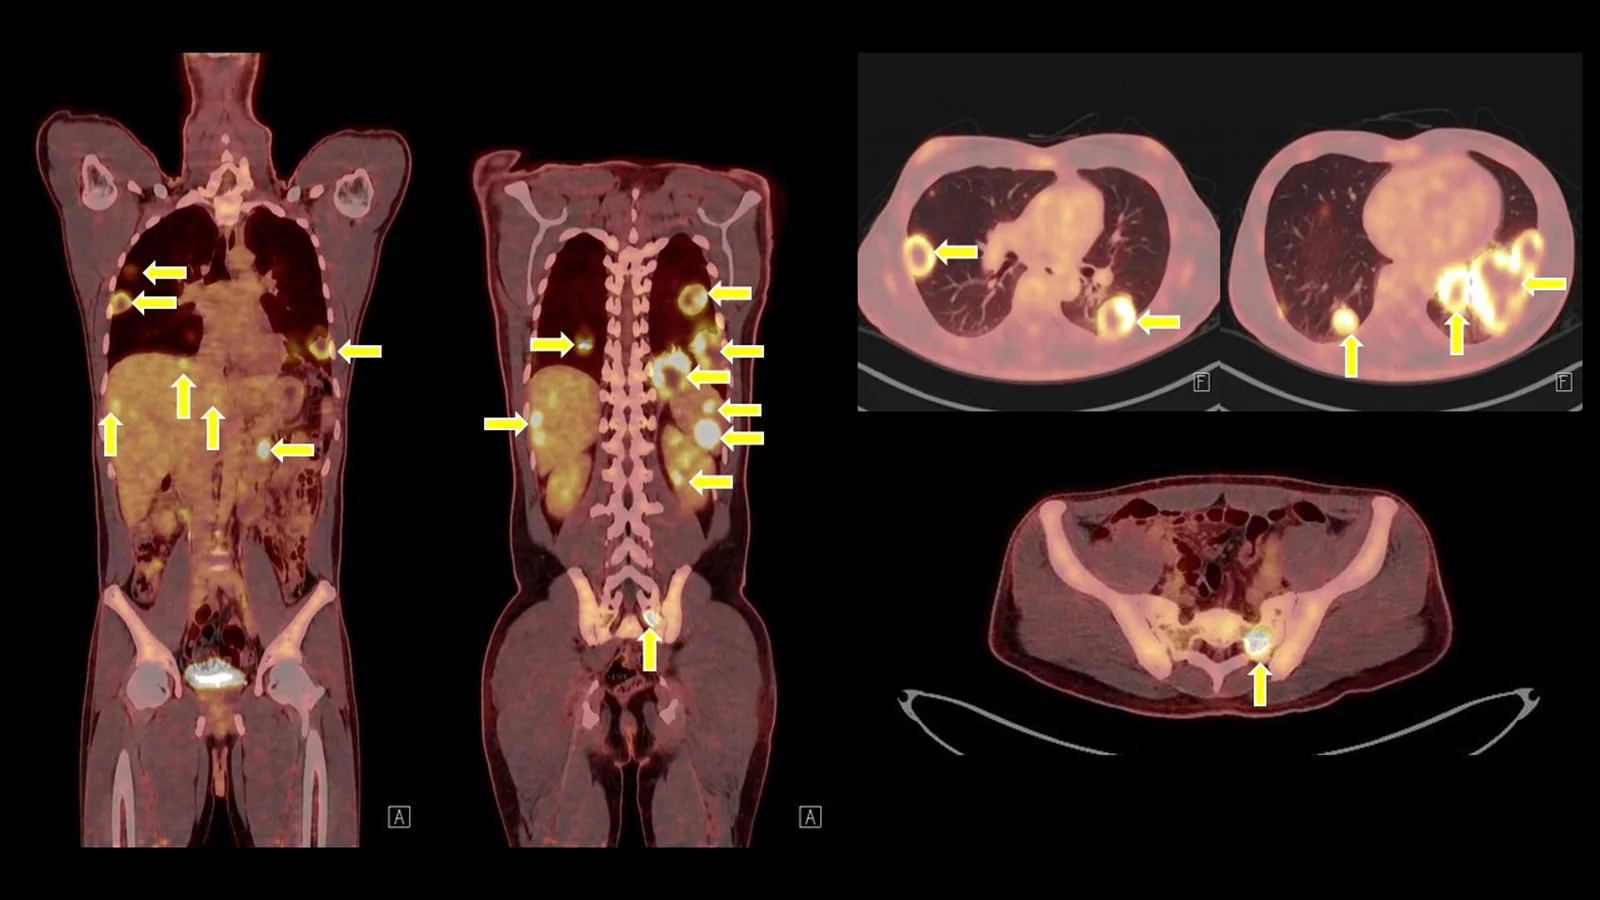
Whole-body FDG PET-CT demonstrating extensive systemic metastases 18F-FDG PET-CT images in coronal and axial views reveal multiple areas of intense hypermetabolism. These hypermetabolic lesions are distributed across the lungs, liver, spleen, bilateral kidneys, and the iliac bone, indicating widespread systemic metastatic disease (yellow arrows) FDG PET-CT: fluorodeoxyglucose positron emission tomography-computed tomography
